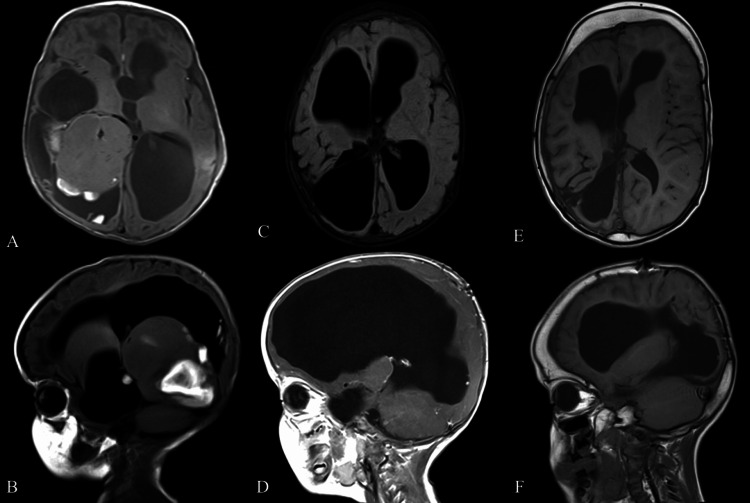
T1 with contrast sequence MRI of the brain: axial (A) and sagittal (B) views showing supratentorial, intraventricular mass (5.2 × 4.4 cm) on the right side, occupying the body of the right lateral ventricle. The mass is heterogeneous in density, including solid and cystic components with lateral, third, and fourth ventricle dilation. Postoperative T1 with contrast sequence MRI of the brain: axial (C) and sagittal (D) views showing extensively enlarged lateral ventricles post-resection of right ventricular GBM. Follow-up: axial (E) and sagittal (F) views of  T1 with contrast sequence MRI of the patient after 5 years showing a reduction in the size of the ventricular system with no evidence of recurrence.
